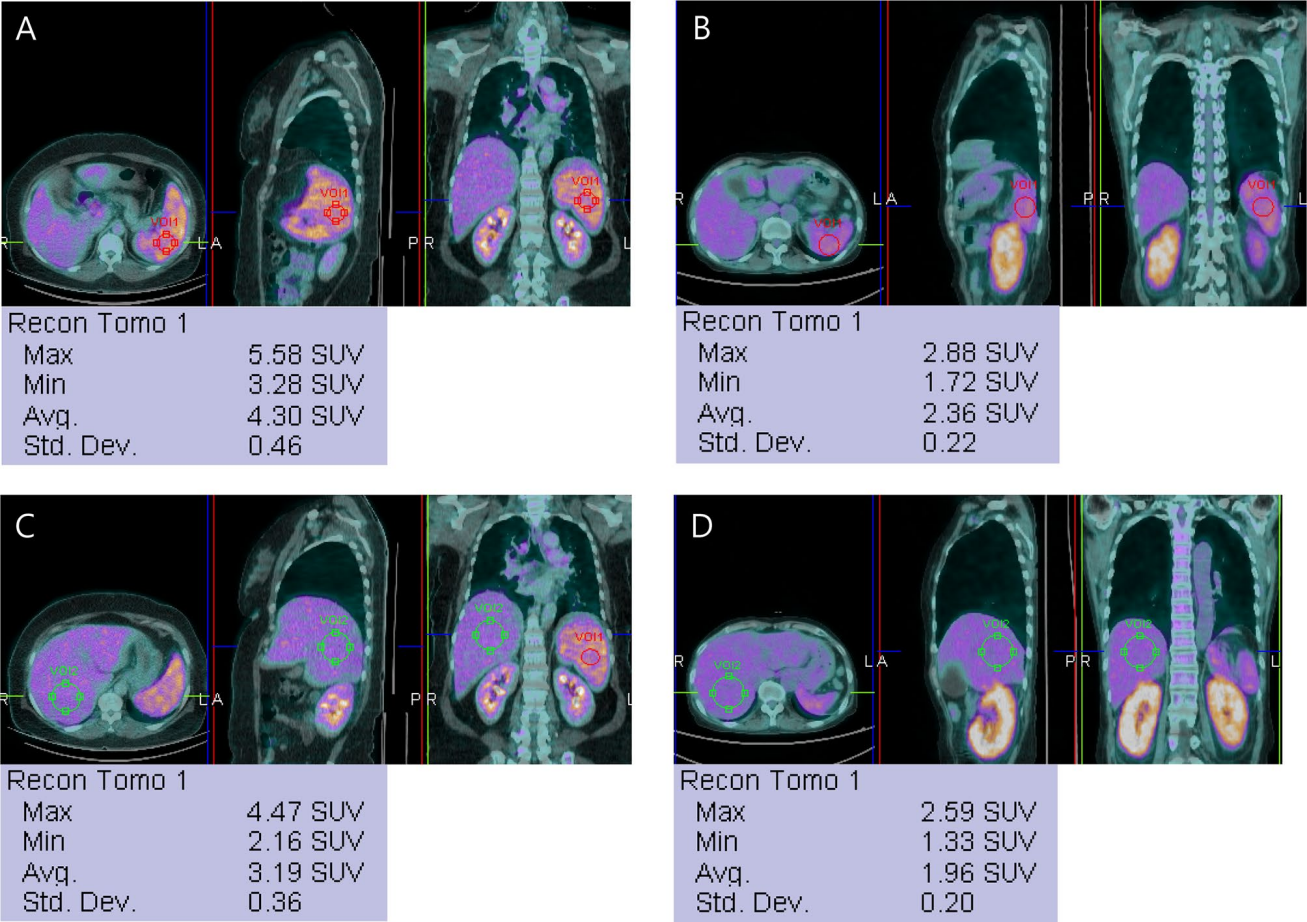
A spherical volume of interest (VOI) 3 cm in diameter is placed on the posterior portion of the spleen. The patient with scrub typhus (A) shows higher SUV_max_ and SUV_mean_ than the patient with SLE (B). The SUV_mean_ of the liver is also measured using a VOI 5 cm in diameter. Like the metabolic parameters in the spleen, the liver also shows higher glucose metabolism in the patient with scrub typhus (C) than in the patient with SLE (D).
